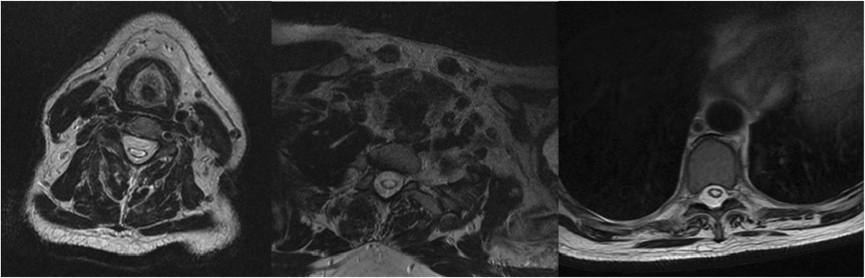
Magnetic resonance imaging of the cervicothoracic spine. Axial T2 fast recovery fast spin echo; the syrinx is visible within the central canal of the spinal cord.
